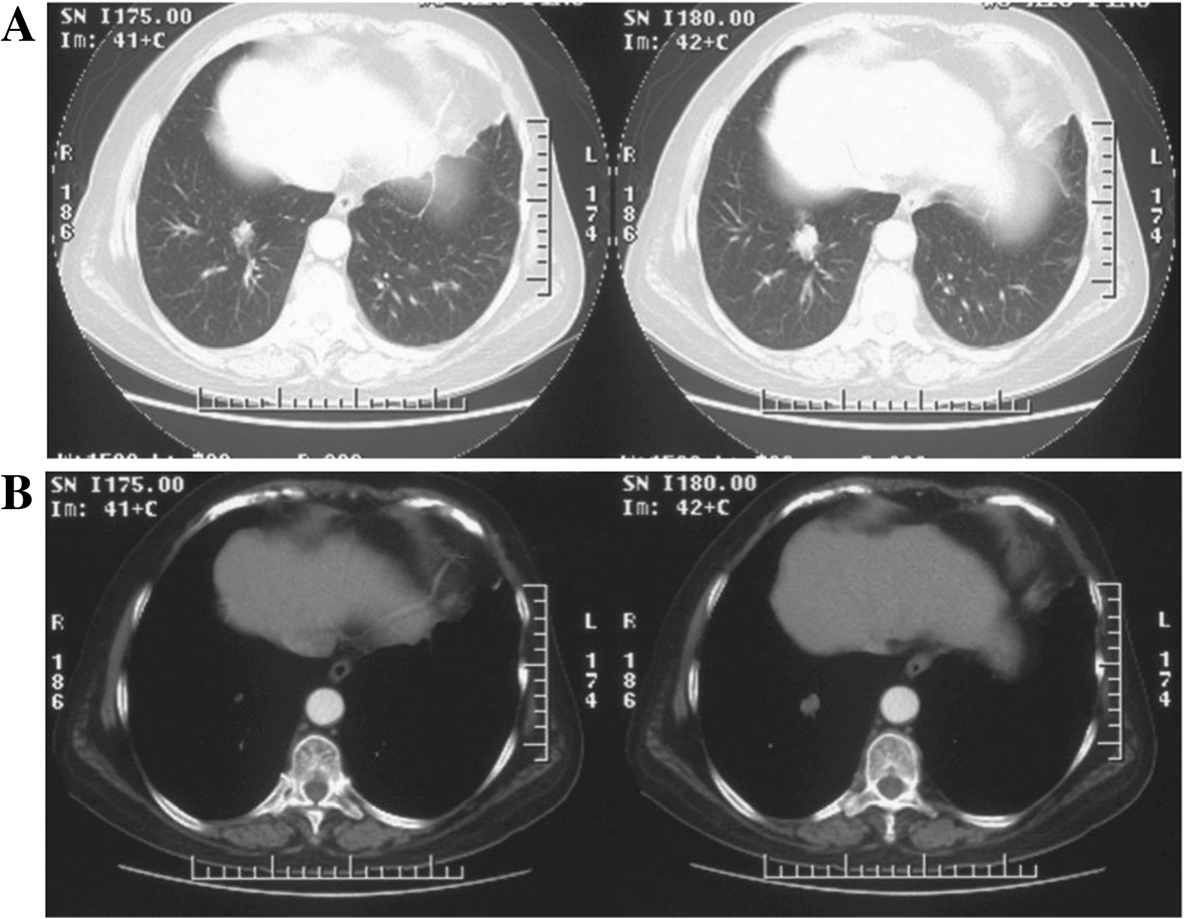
Computed tomography scans of chest: A, B) tumor-like mass with blurred contour and irregular margin shown in lung and mediastinal windows.
